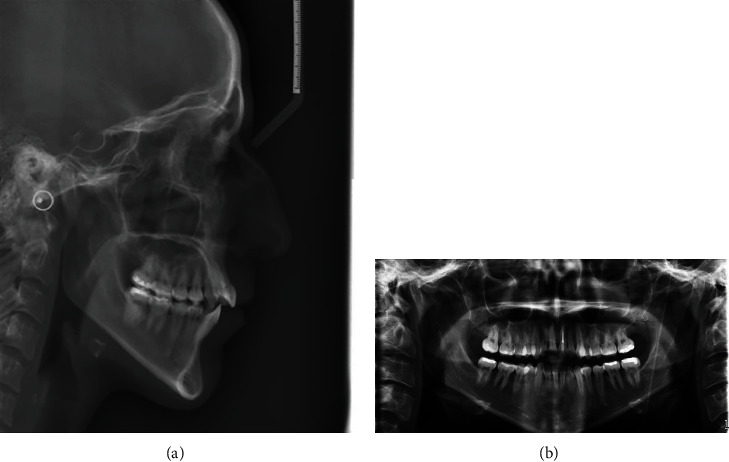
(a, b) Initial radiographic records.
